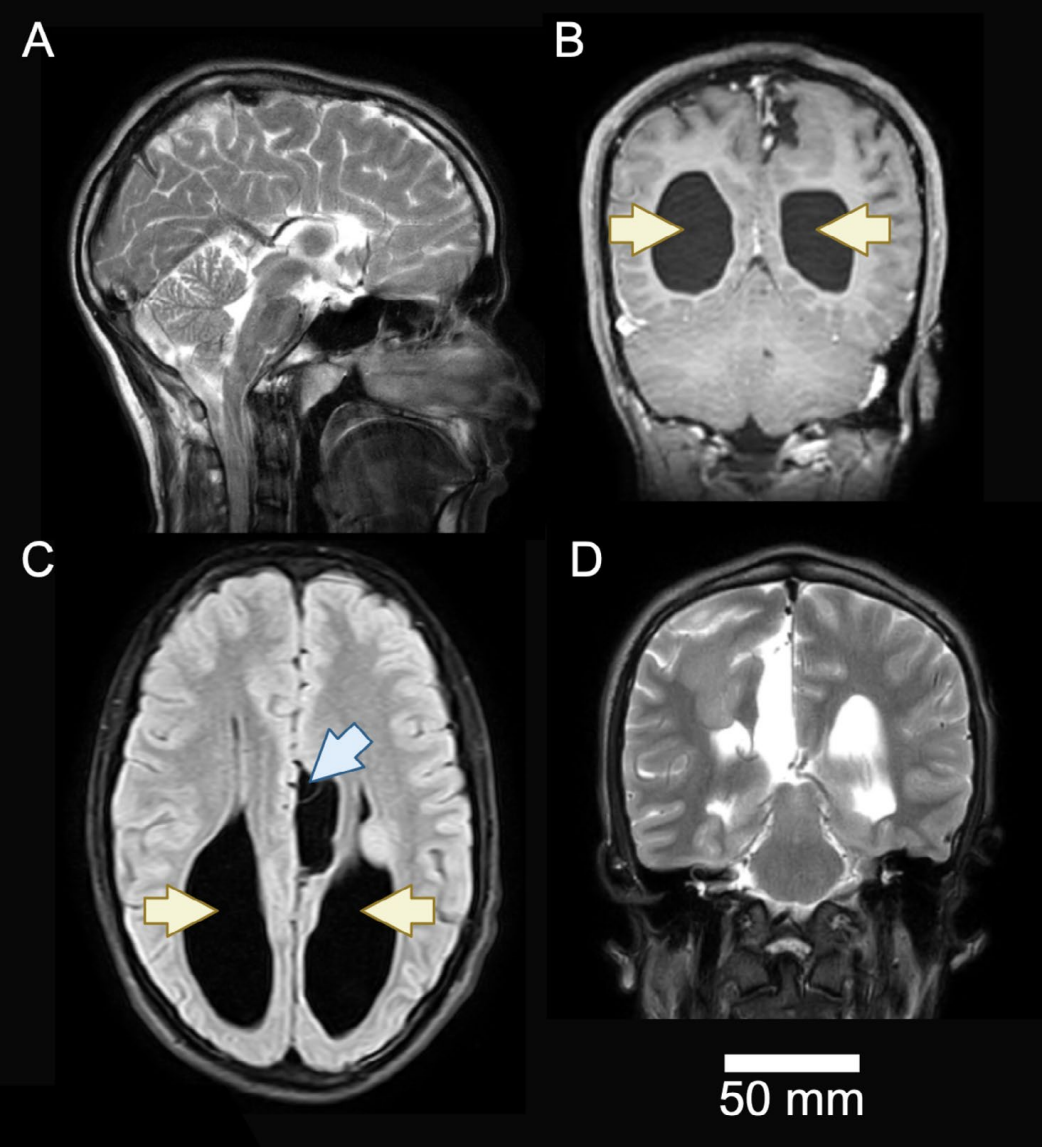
MRI images of the patient with agenesis of corpus callosum. (A) T2‐weighted sagittal MRI, (B) T1‐weighted coronal MRI, (C) FLAIR axial, (D) T2‐weighted coronal MRI (at the level of anterior horns). Yellow arrows indicate enlarged lateral ventricles, while the blue arrow points to an interhemispheric cyst.
